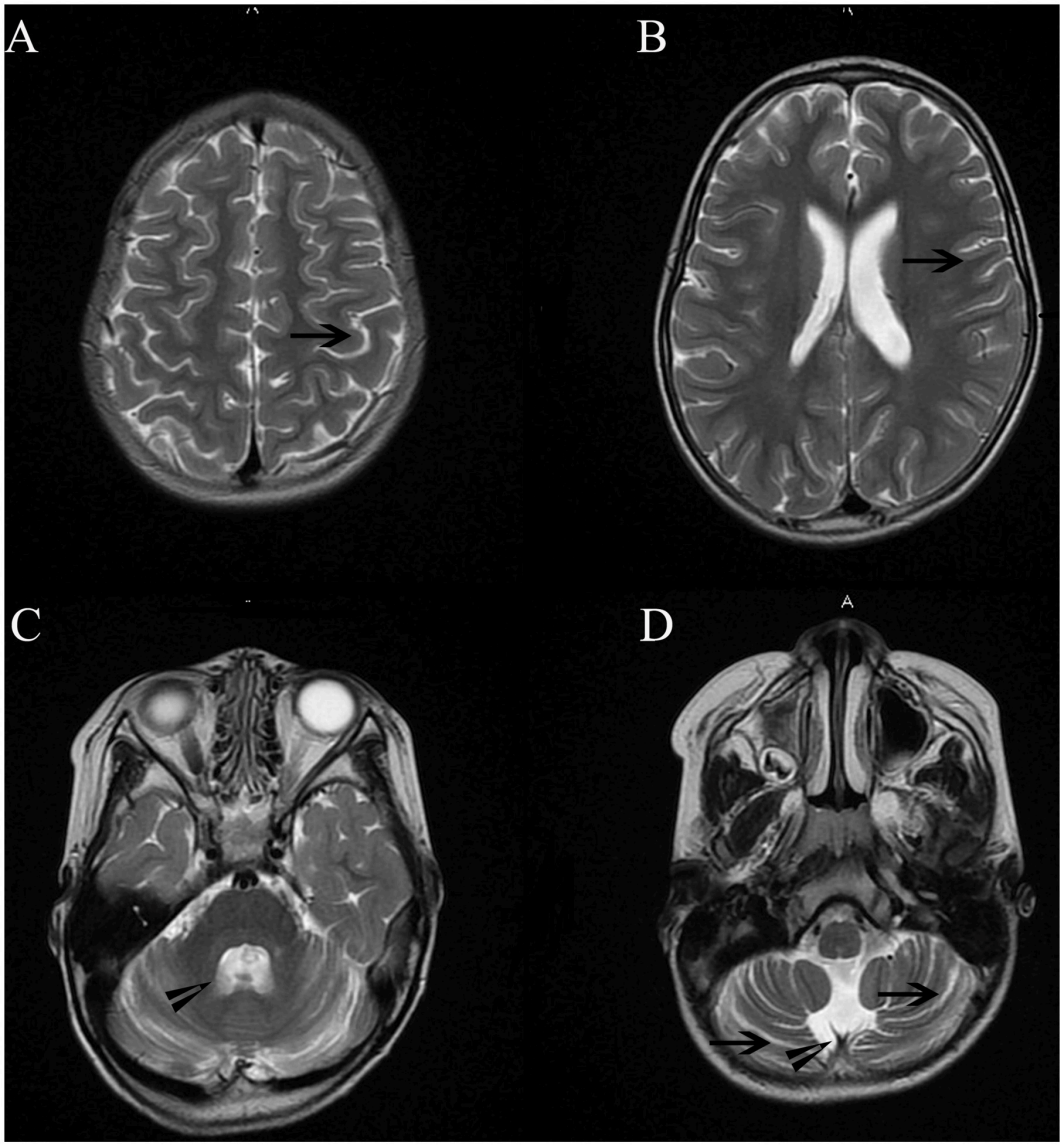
Cranial MRI showed mild atrophic changes in bilateral hemisphere and cerebellum. Ventricular systems were generally enlarged. Cerebral sulcus and fissures widened in bilateral hemisphere and cerebellum. Long arrowheads in Figure (A,B,D) showed widened sulcus and fissures in hemisphere and cerebellum. Short arrowheads in (B,C) showed enlarged the fourth ventricle and cisterna magna.
